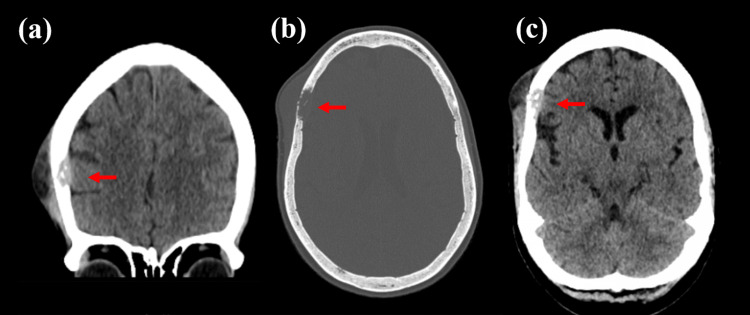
Computed tomographic scan of the head showed calvarial TB. (a) Coronal CT scan demonstrates a peripherally enhancing epidural collection at the right parietal region with overlying destructed right parietal bone not crossing the suture and sub-galeal lesion. (b) Axial bone window demonstrates the disintegration of the right parietal bone. (c) Axial CT image demonstrating the outer and interior table defect with extradural collection.
